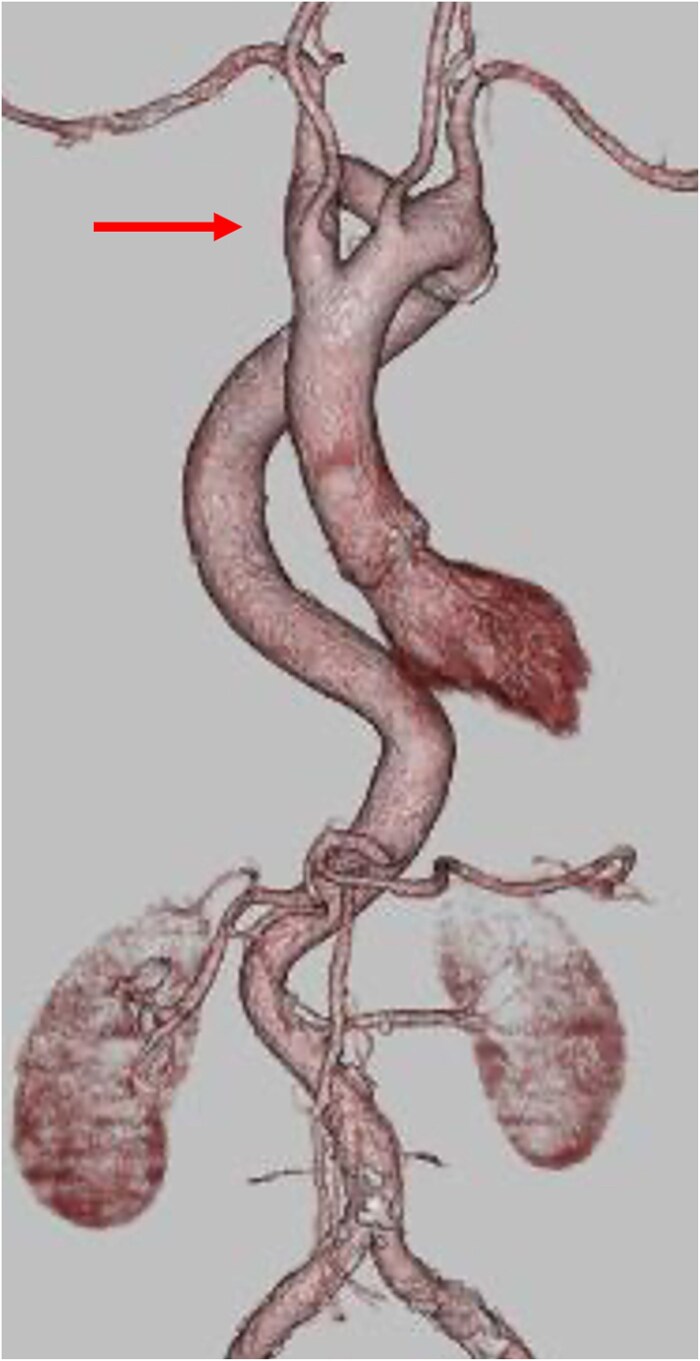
Three-dimensional CT reconstruction showing the double aortic arch (arrow) compressing the trachea.
